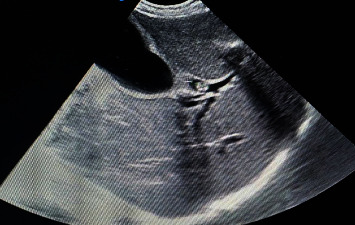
Ultrasound image of ascaris worm in the common bile duct, transverse section.
